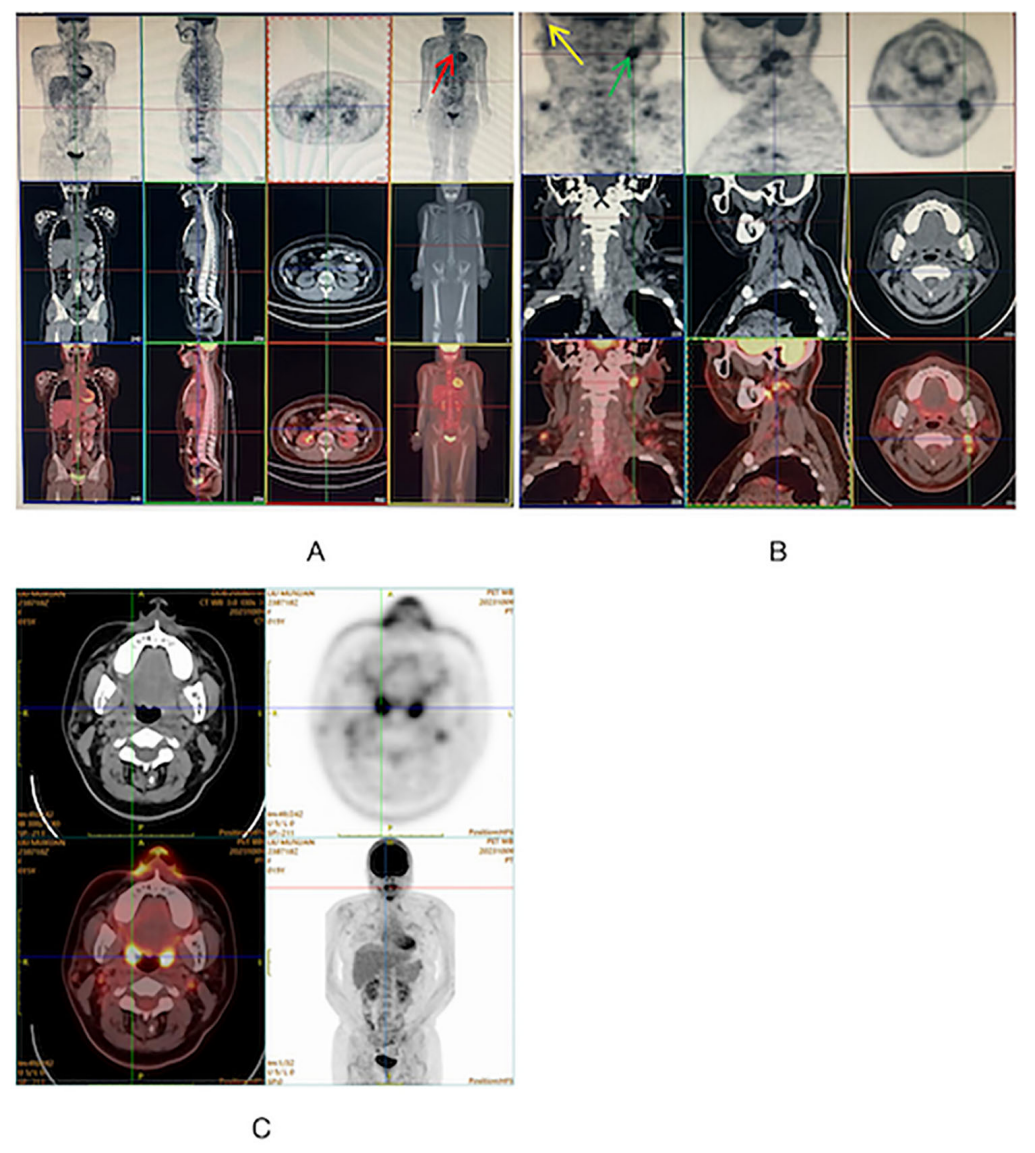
(A, B) FDG-PET on admission showing diffuse uptake in mediastinum supradiaphragmatic lymph nodes (Red), left supraclavicular lymph node (Yellow) and right cervical lymph nodes (Green). (C) FDG-PET at 11 months after diagnosis showing disappearance of abnormal signals.
